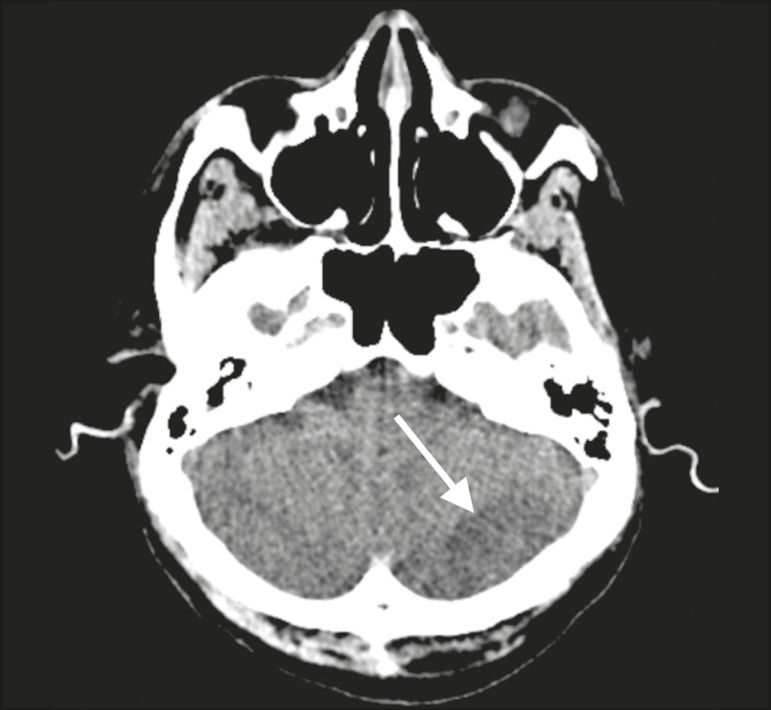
Case 2. Brain CT of an 81-year-old man with COVID-19 showing an acute ischemic stroke in the left cerebellar hemisphere, in the posterior inferior cerebellar artery territory (arrow).
